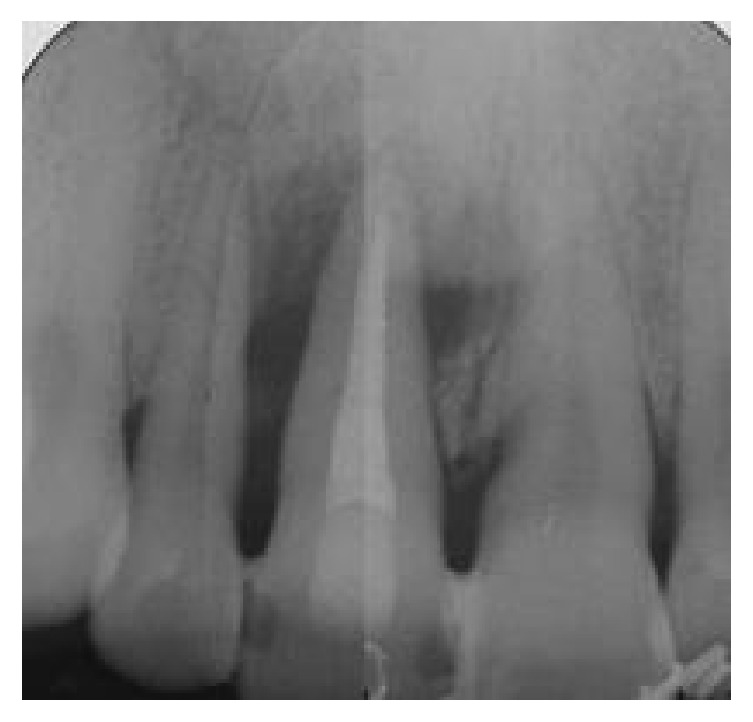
Intraoral periapical radiograph showing bone loss around the right central incisor and involving the mesial alveolar wall of the right lateral incisor.
